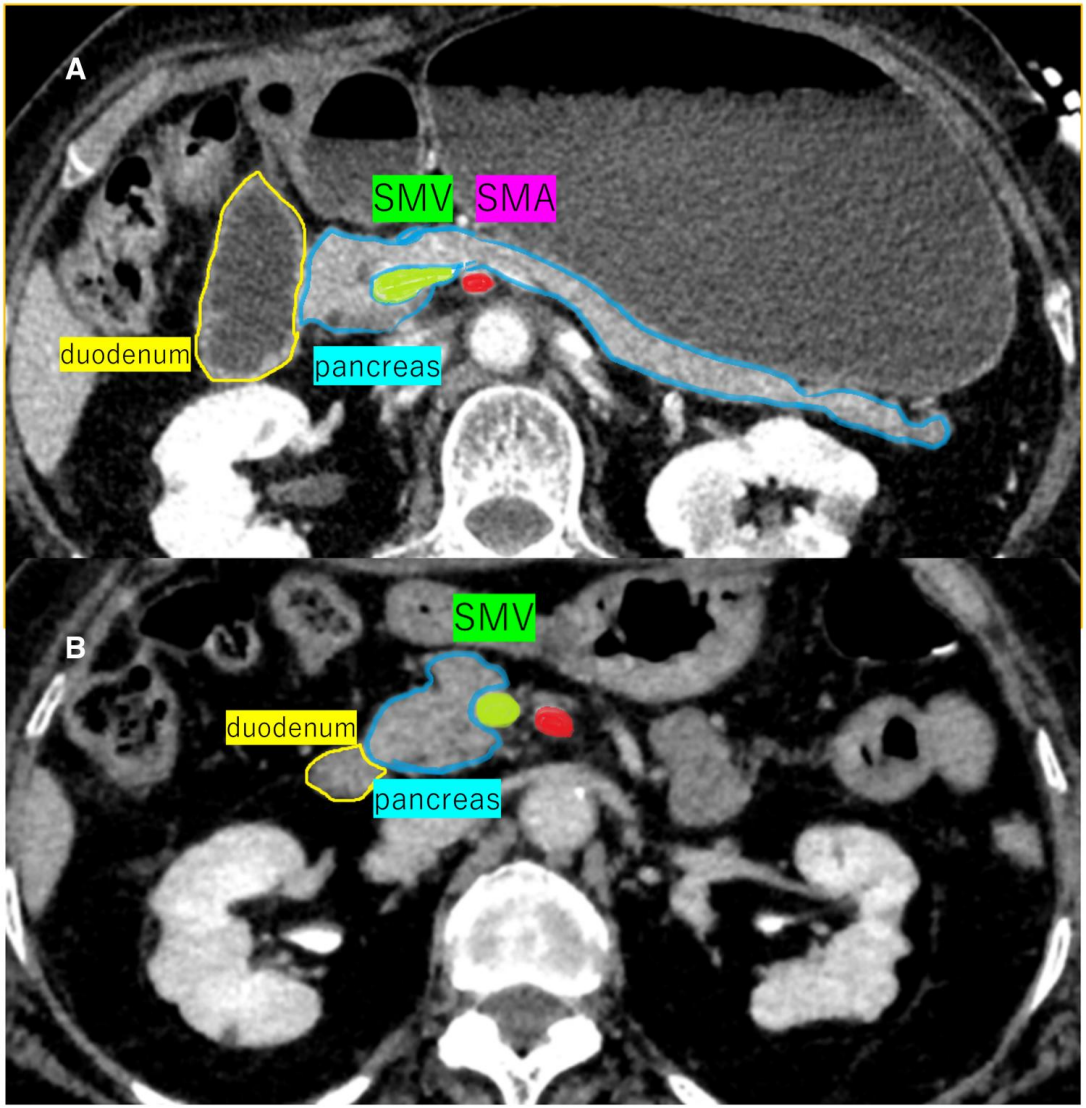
The upper CT image (axial view) is at the L2 level, and the image was obtained at the time of haematemesis. The image shows that the duodenal-jejunal junction is deviated to the right (A). The lower CT image (axial view) is at the same level and was obtained at the time of spontaneous resolution of the intestinal malpositioning (B). Comparing both CT images, the anterior pararenal space organs, such as the duodenum and pancreas, are shifted to the right when the position of the duodenal-jejunal junction is deviated to the right. Abbreviations: SMA = superior mesenteric artery; SMV = superior mesenteric vein.
